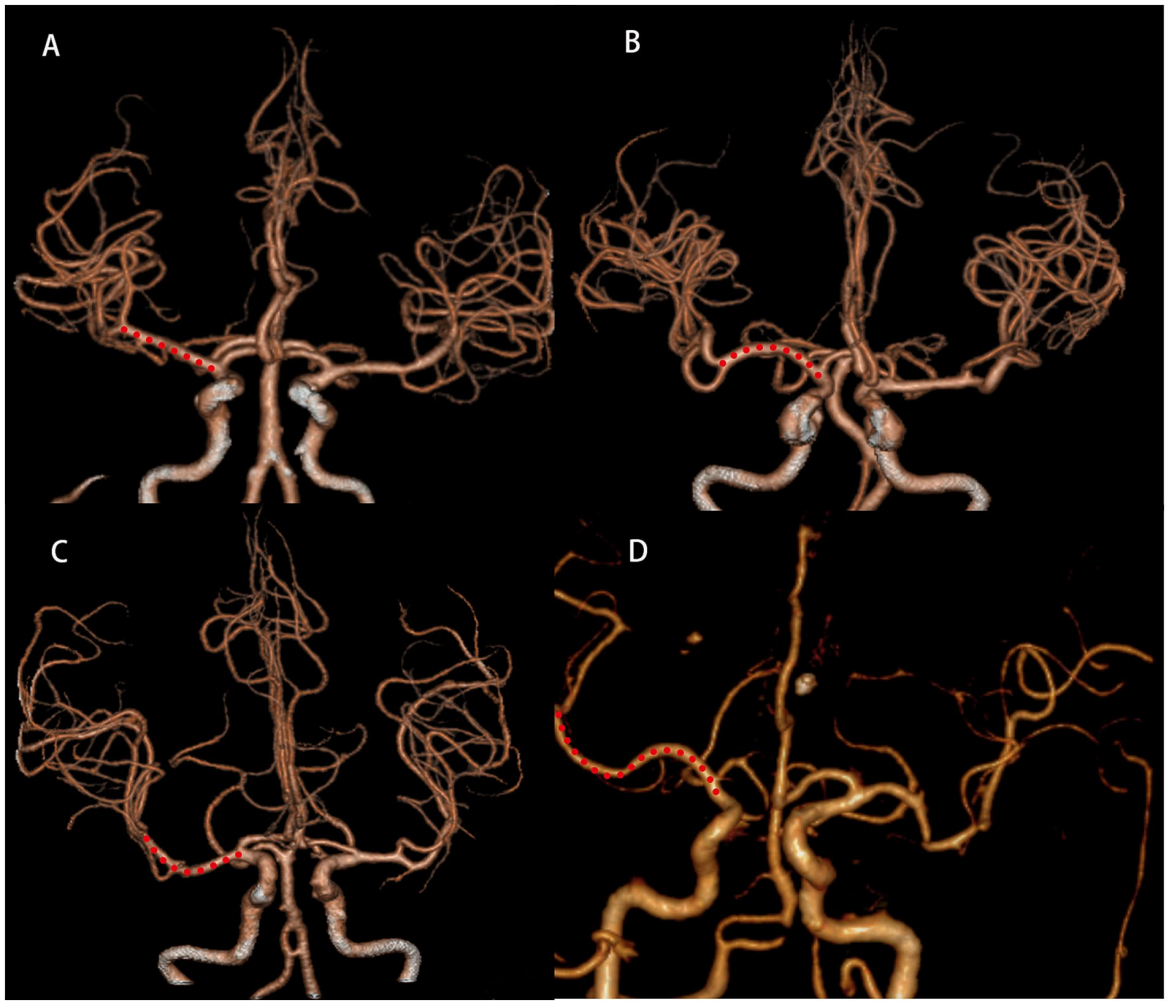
Shape of MCA from three-dimensional reconstructed image of CTA. Straight (A), inverted U-shaped (B), U-shaped (C) and S-shaped MCAs (D). MCA, middle cerebral artery; CTA, computed tomography angiography.
